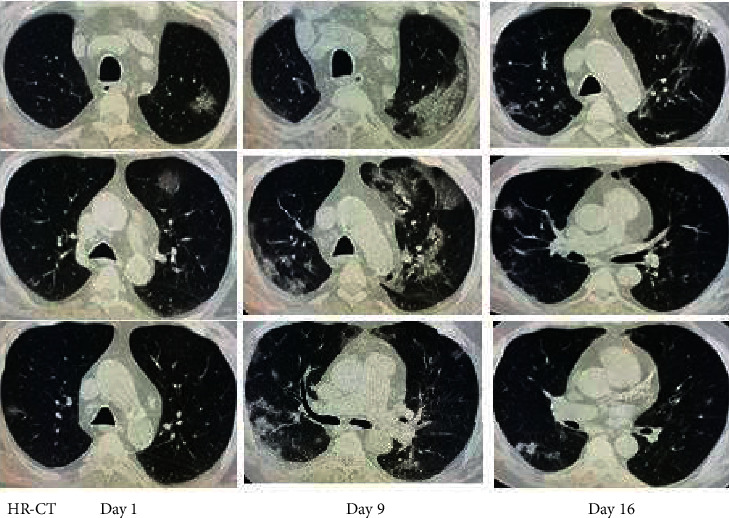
HR-CT findings on day 1 with few focal ground-glass opacifications (GGO), day 9 with GGO dissemination, and day 16 with substantial improvement of the lung parenchyma.
